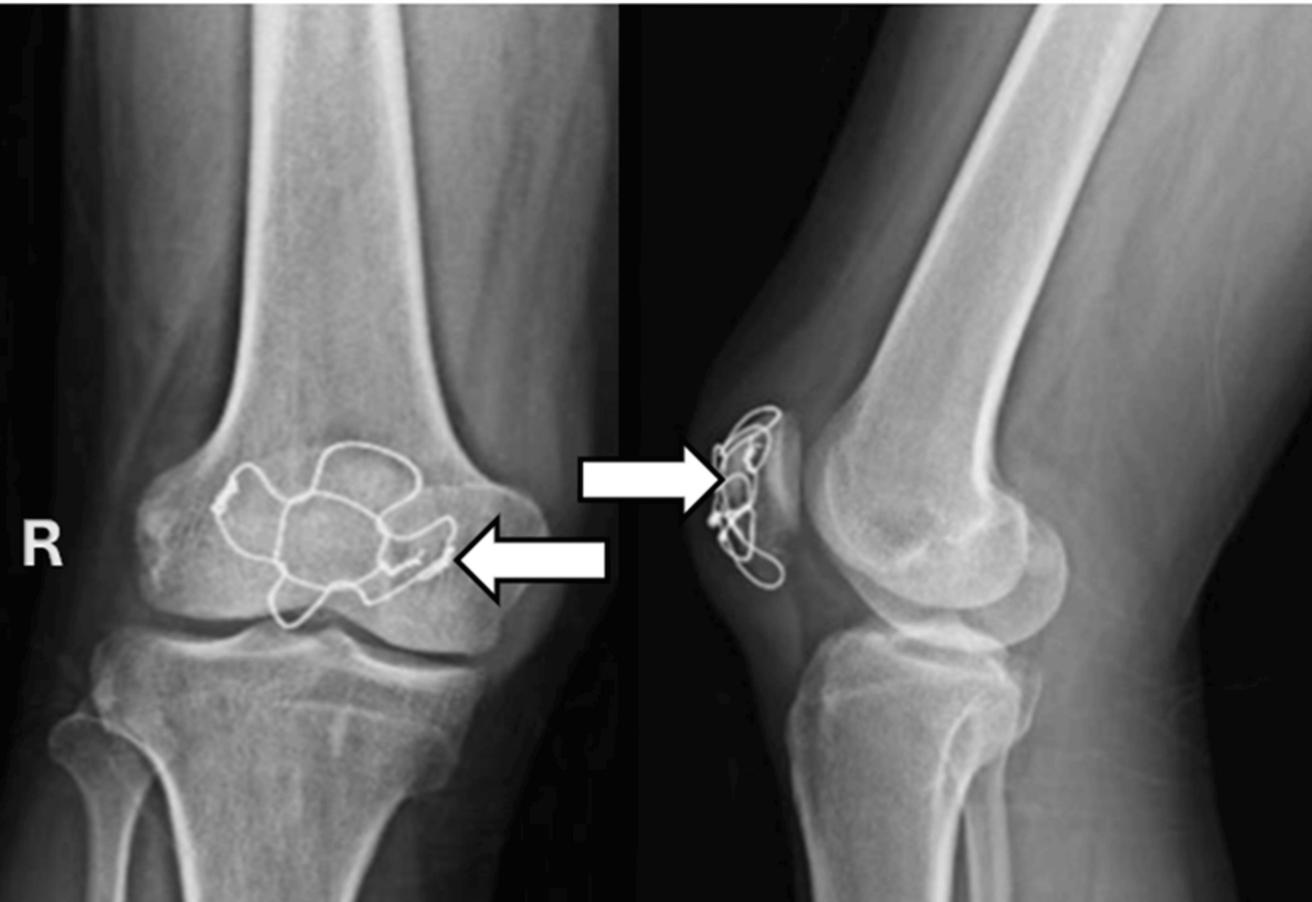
Radiograph (anteroposterior and lateral views) at three months showing union.
